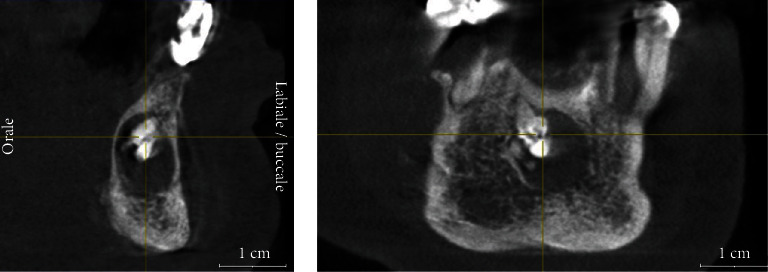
CBCT scan performed in 2020, showing the enlargement of the lesion compared to the first CBCT scan.
